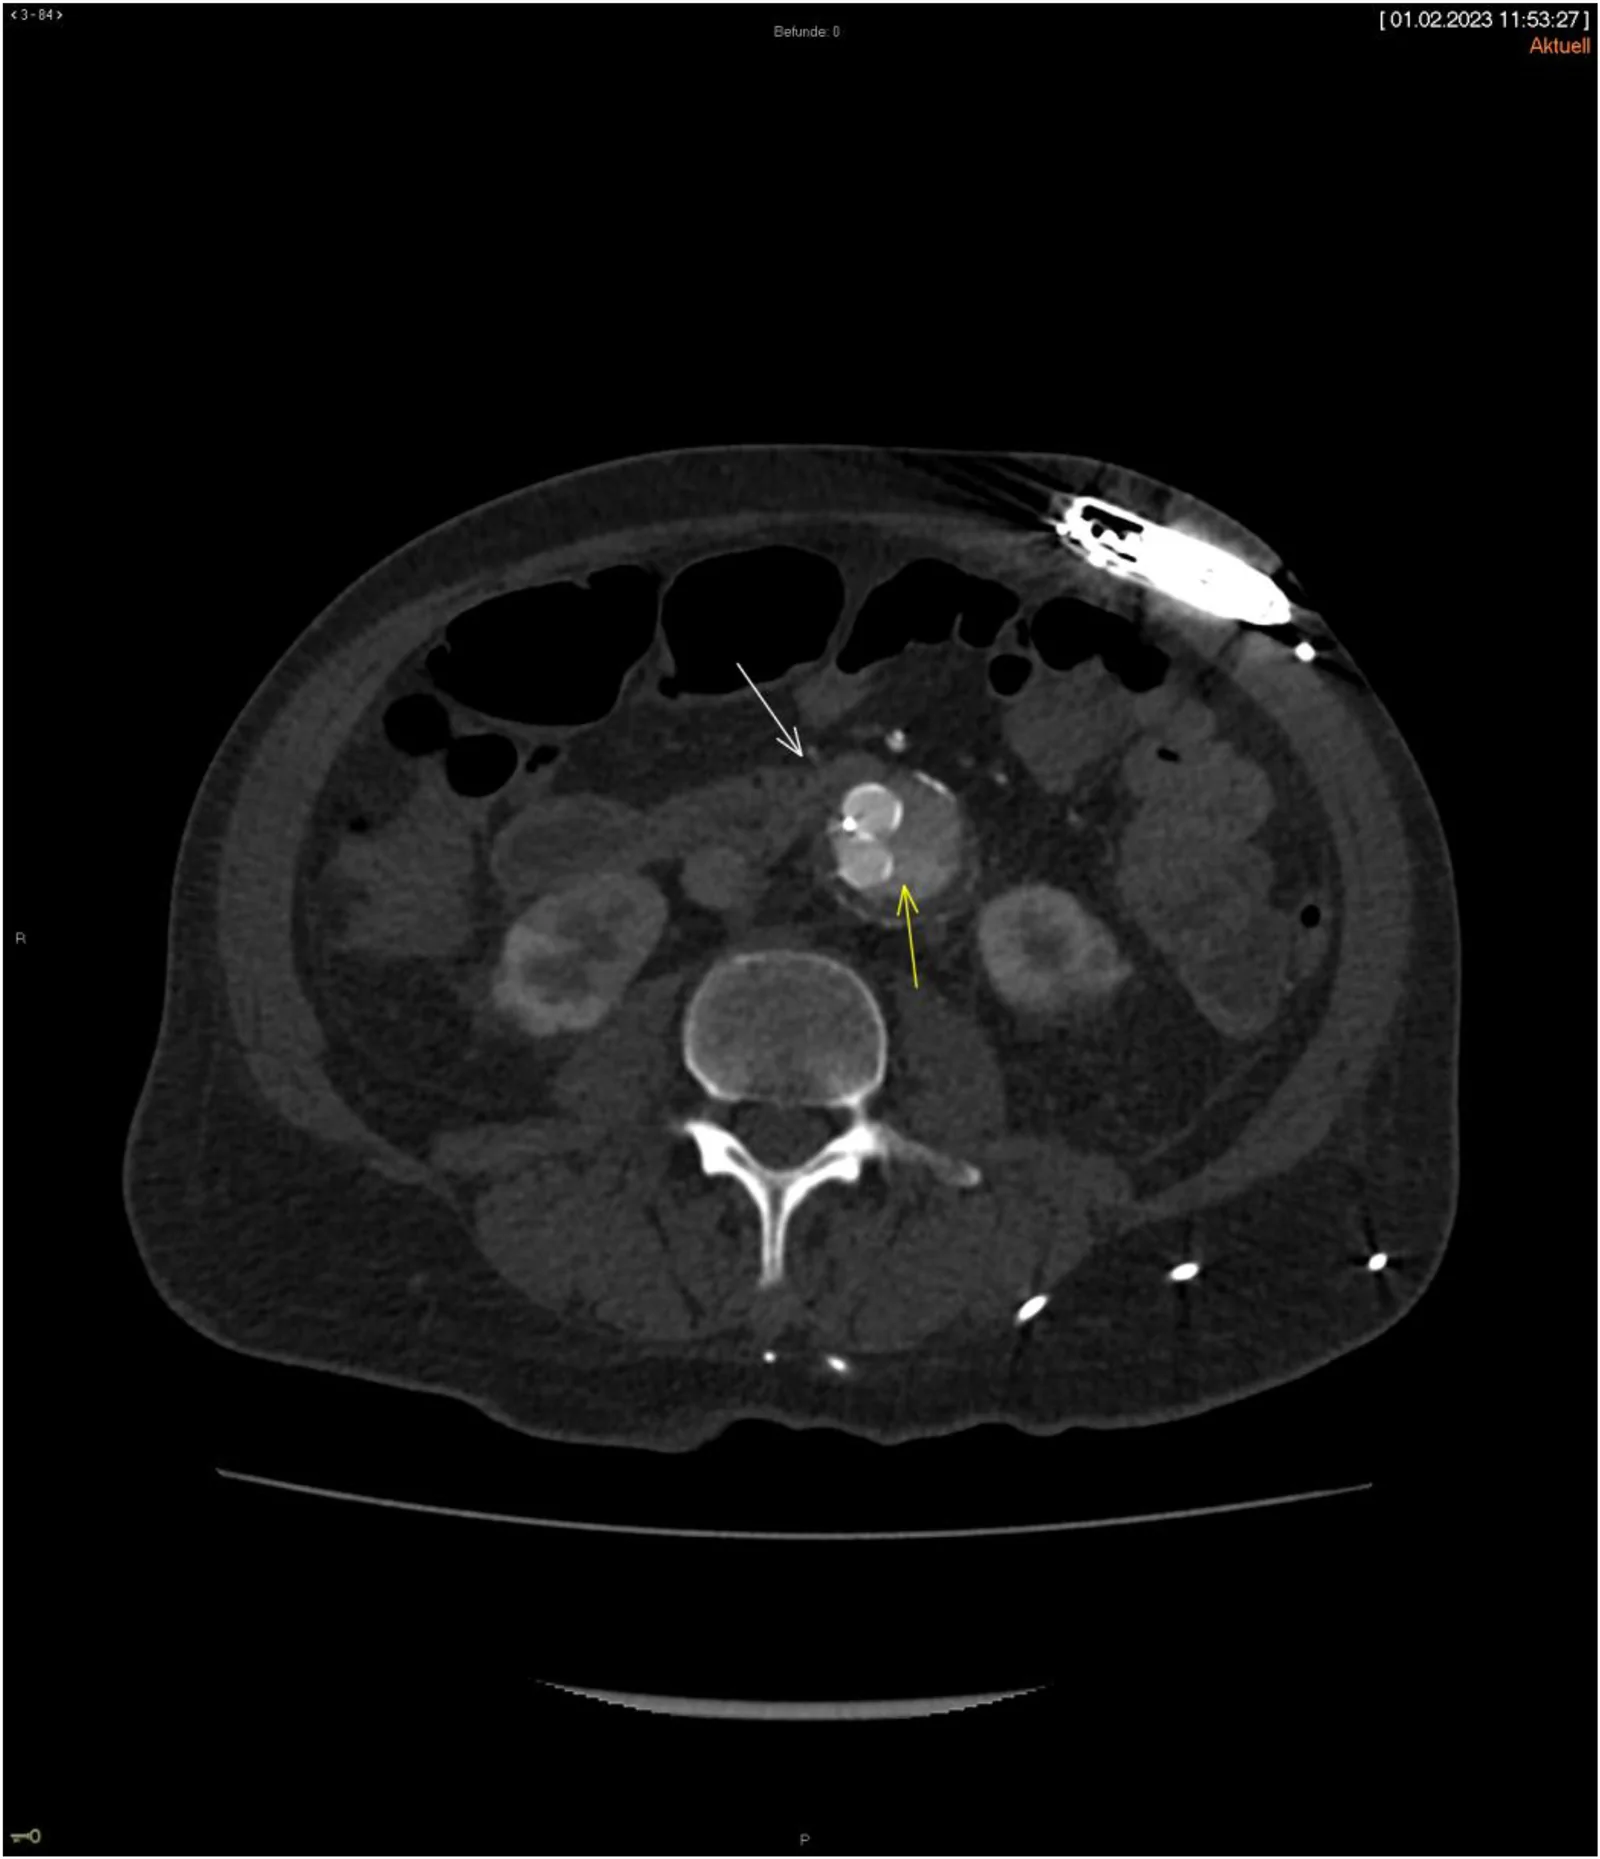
Post-EVAR axial contrast-enhanced CT image obtained at the referring institution demonstrating an endoleak within the aneurysm sac (yellow arrow). Focal narrowing of the adjacent third portion of the duodenum is also present (white arrow), without convincing upstream duodenal dilatation on the available image.
